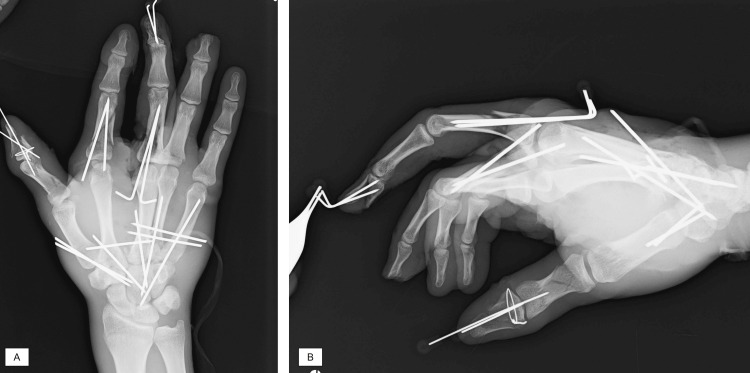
Postoperative radiographs of the right hand Postoperative oblique view (A) and lateral view (B) of the right hand demonstrating stabilization of multiple metacarpal and phalangeal fractures, as well as the third CMC joint.
